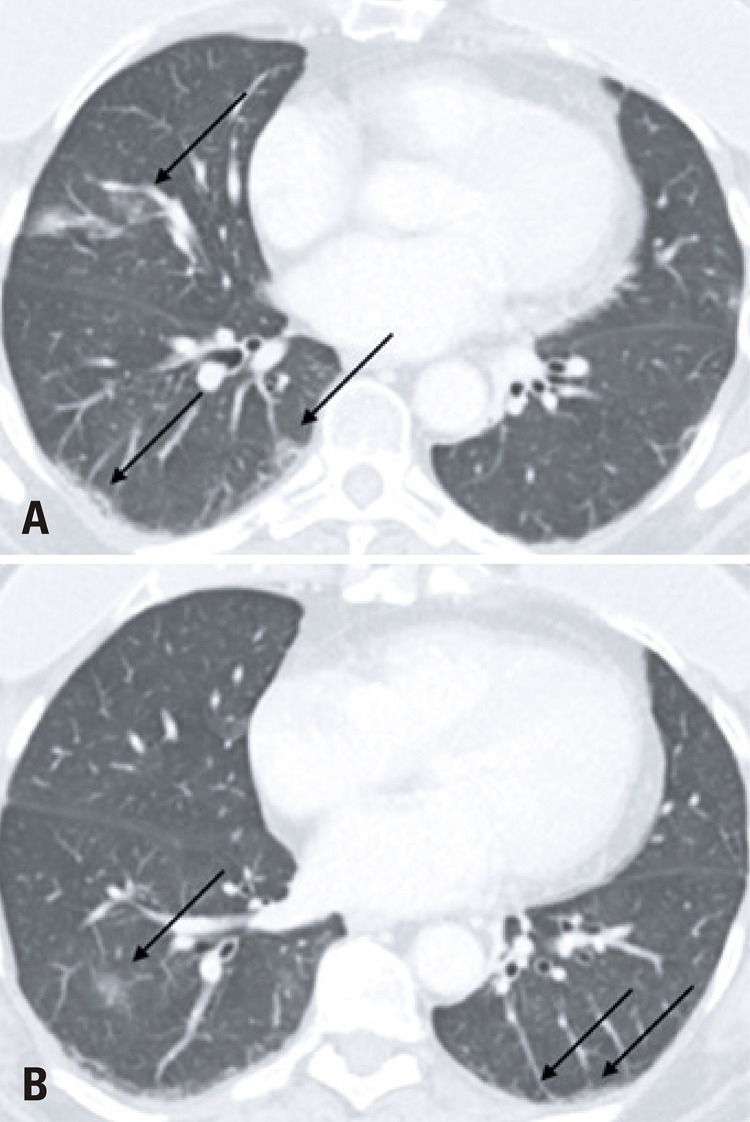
Axial images (A and B) of the lung basis in an abdomen computed tomography show subtle ground glass opacities with some septal thickening, which possibly represented incipient COVID-19 changes (arrows)
